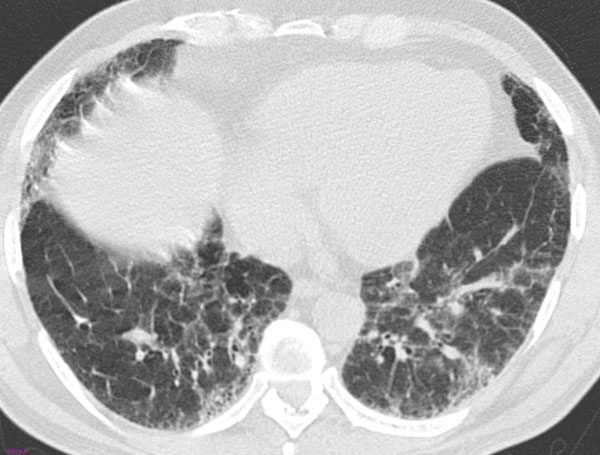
Biopsy-proved UIP with an HRCT pattern inconsistent with UIP. There are some patchy ground-glass and peripheral reticular opacities. which are more suggestive of nonspecific interstitial pneumonia (NSIP).
